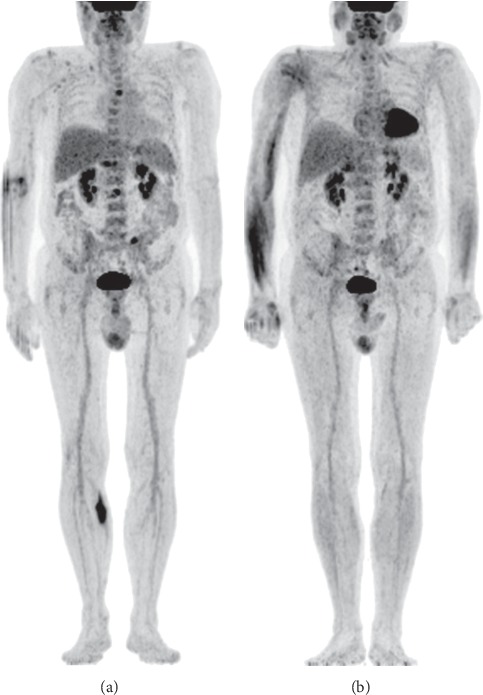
F18-FDG PET/CT performed at staging showed an intensely hypermetabolic lesion in the right leg and revealed three additional vertebral hypermetabolic lesions in D5, L2, and S1 (a). After chemotherapy, the hypermetabolic lesions in the right leg, D5, and L2 had completely disappeared, with persistence of moderate metabolic activity in the left sacral lesion (DS = 3) (b).
